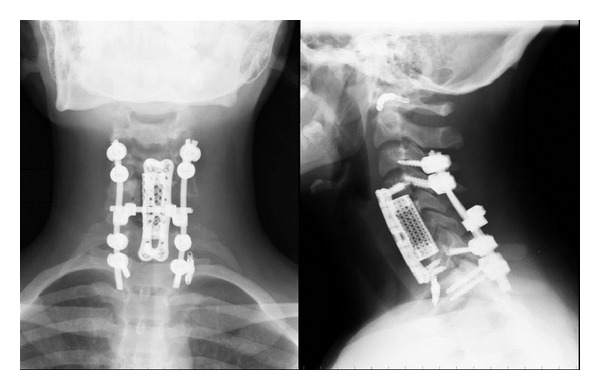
Postoperative cervical spine radiographs.
